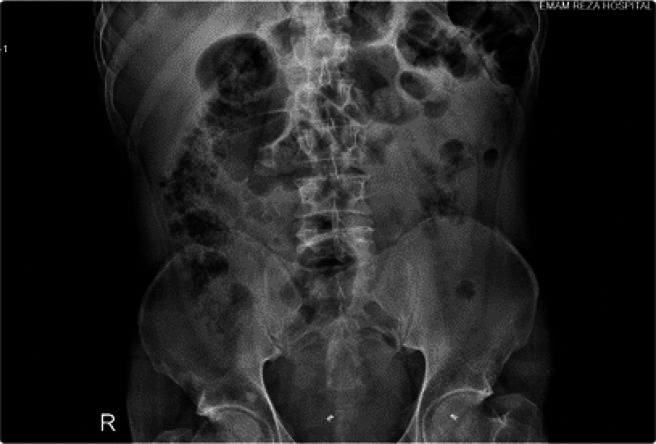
Plain abdominal X-ray showing distention at the proximal and air-free level at the distal loop
